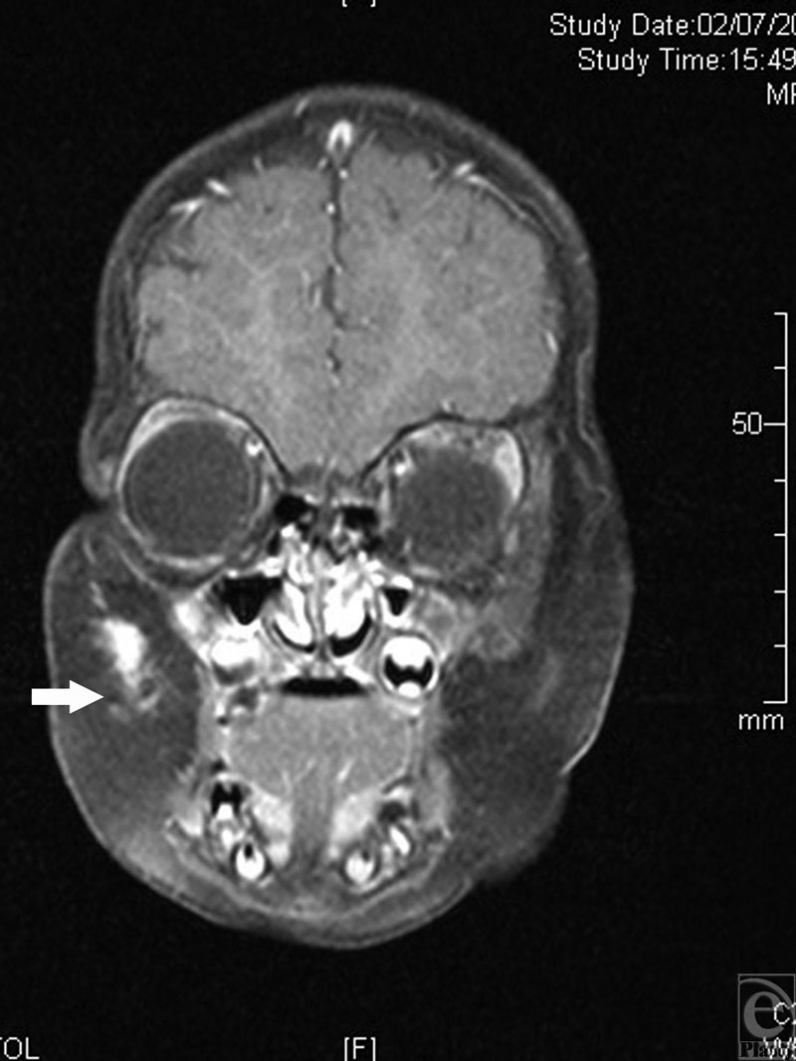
Magnetic resonance imaging study performed at the age of 3 months. A hyperintense fibrotic mass in the subcutaneous layers of the right cheek, penetrating to the upper lip is demonstrated (arrowhead).
